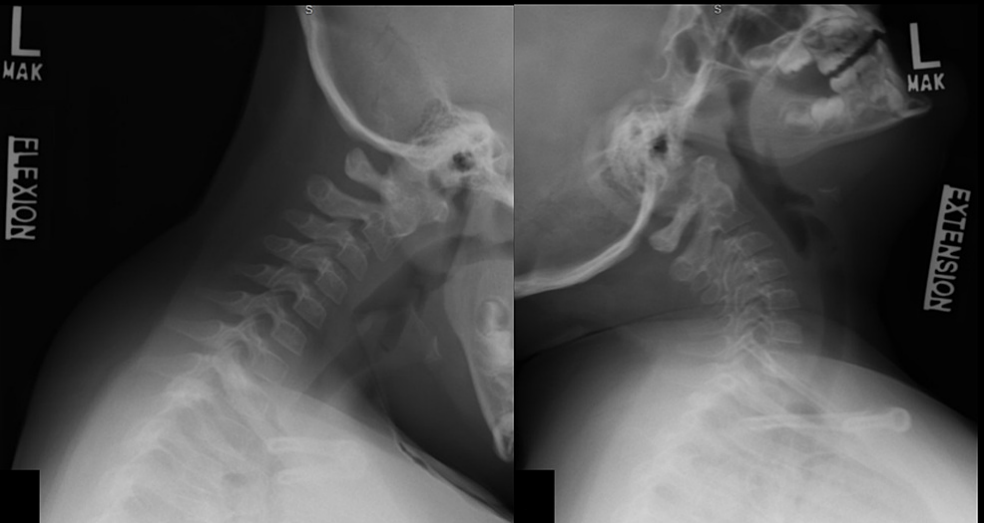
Lateral views of flexion-extension plain films of the cervical spine demonstrating no evidence of instability at the C1/2 junction after removal of hardware.
